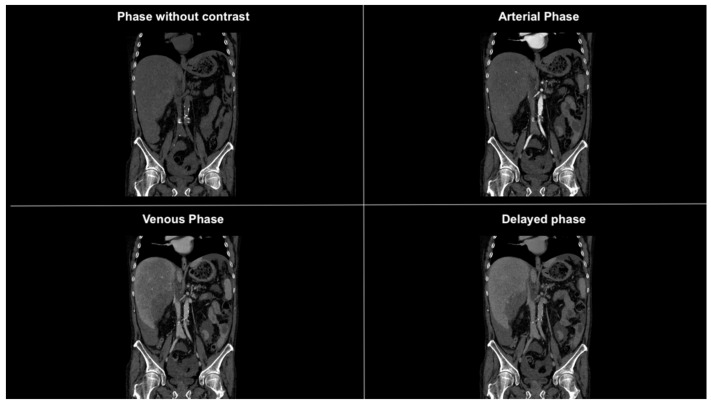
Coronal CT images in different phases showing subdiaphragmatic fluid collection bilaterally, within the paracolic gutters, and prominently around the liver. The fluid collection is suggestive of hemoperitoneum.
